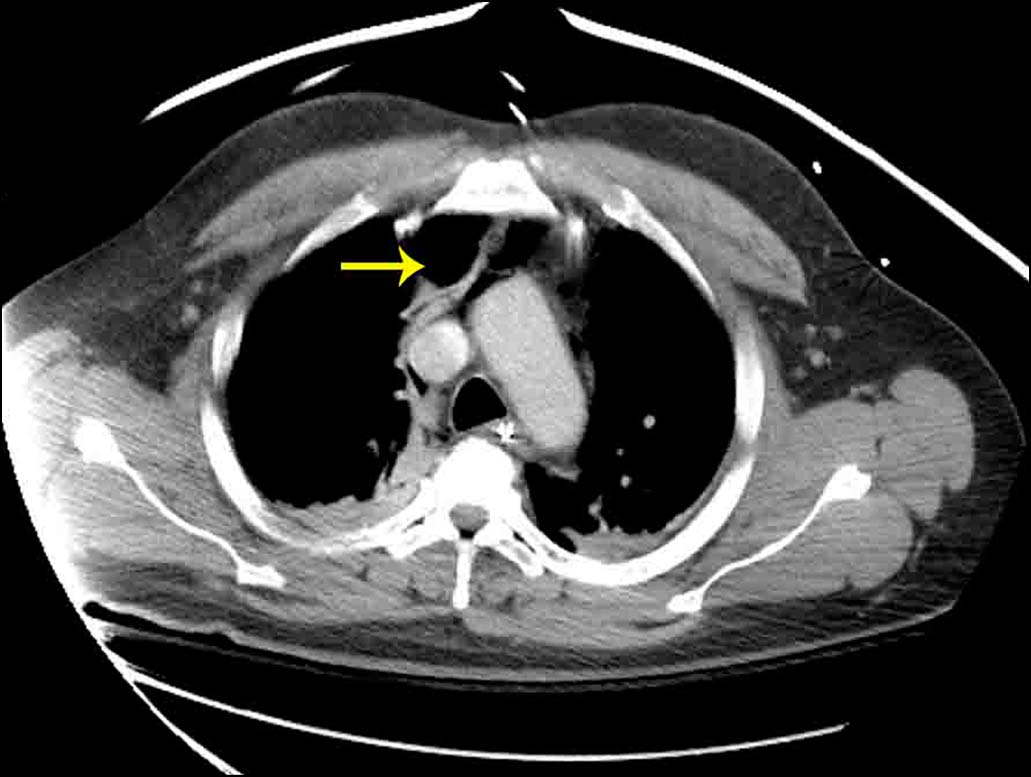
CT showing pneumomediastinum (arrow) following cryoablation. CT, computed tomography.
